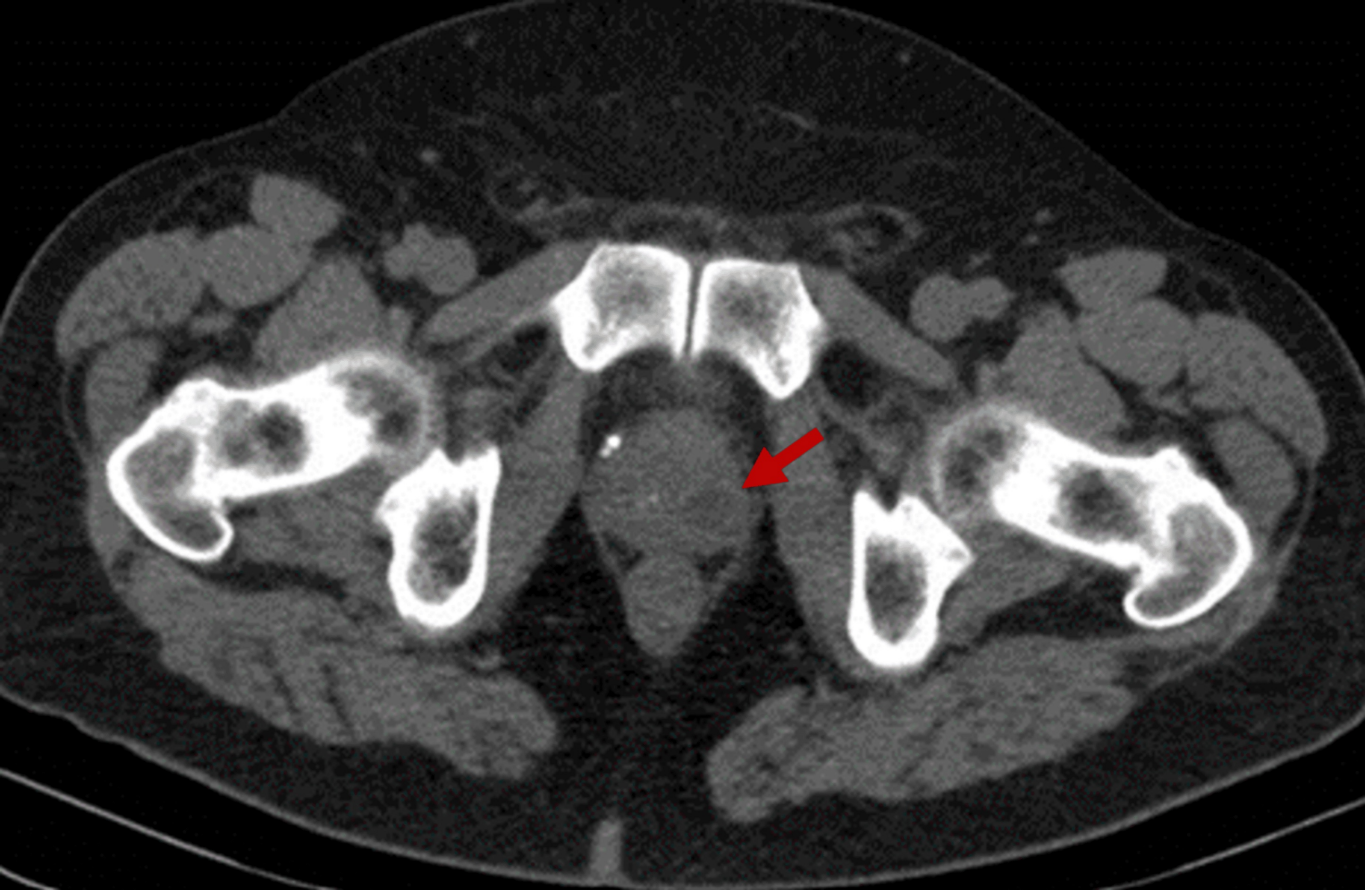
Abdominopelvic CT scan demonstrating a fluid-density lesion in the left transition zone of the prostate suggestive of a prostatic abscess (arrow).
